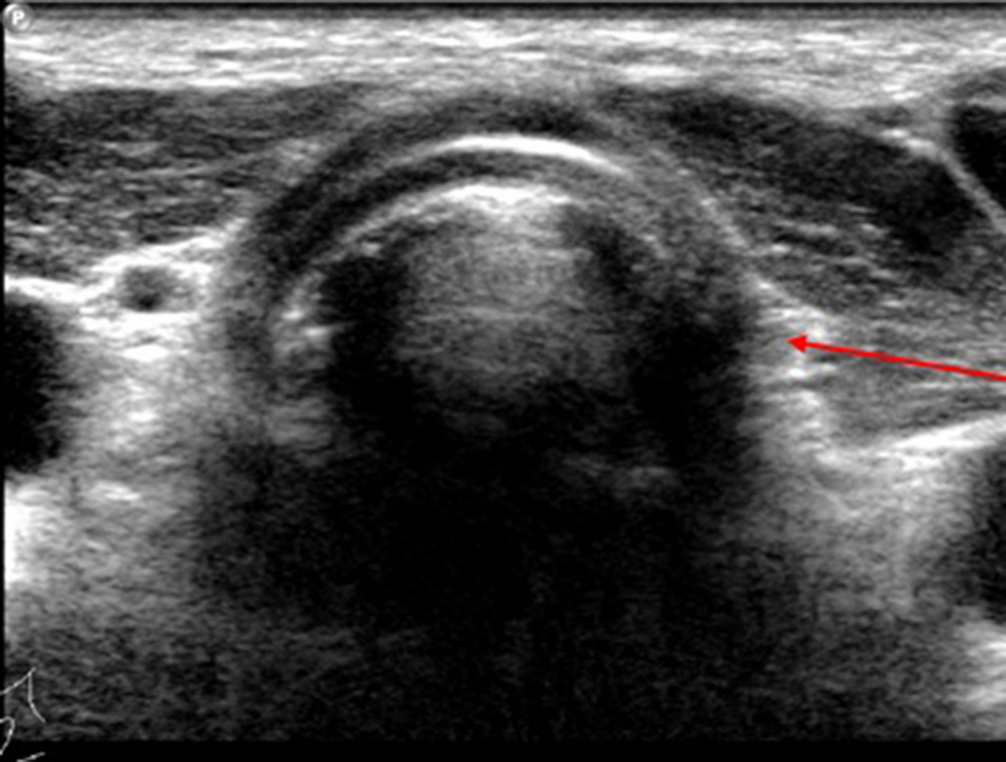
Post-operative neck ultrasound demonstrating trachea and strap muscles. The brightly
